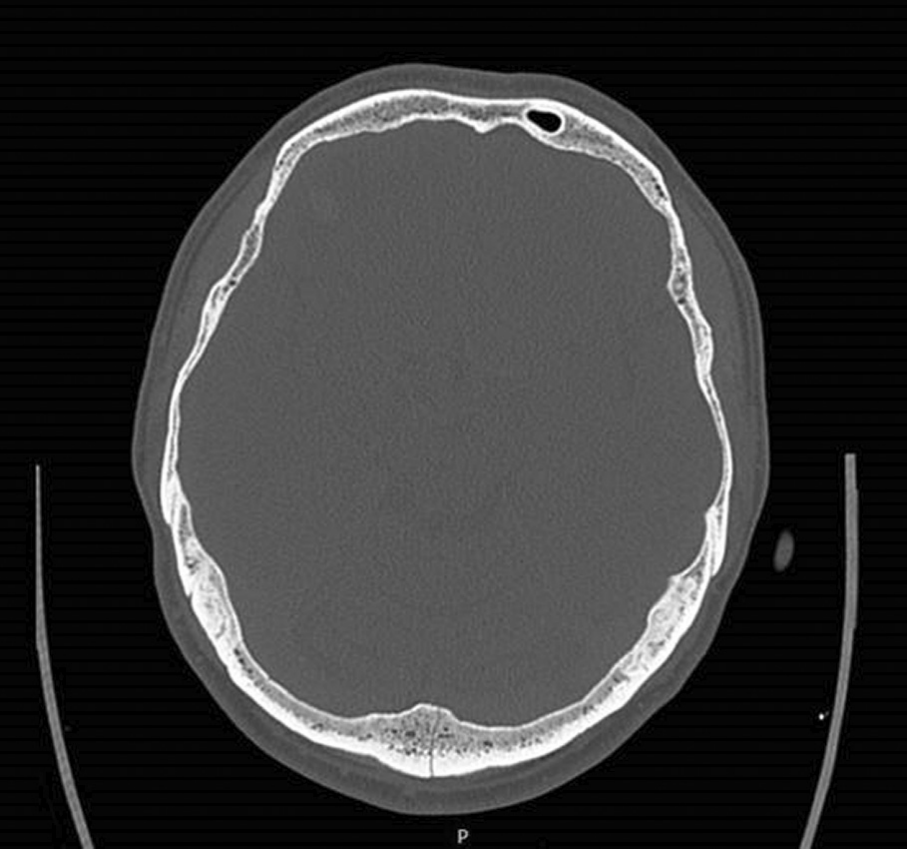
Computed tomography of the head, axial view
